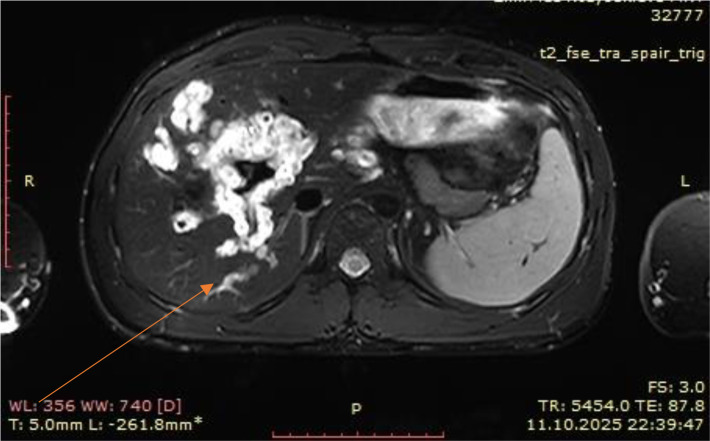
Extensive plexiform neurofibroma of the right lobe of the liver in the studied patient on 3 Tesla MRI (5 mm slice thickness) in Fat Saturation (FS) regime.
